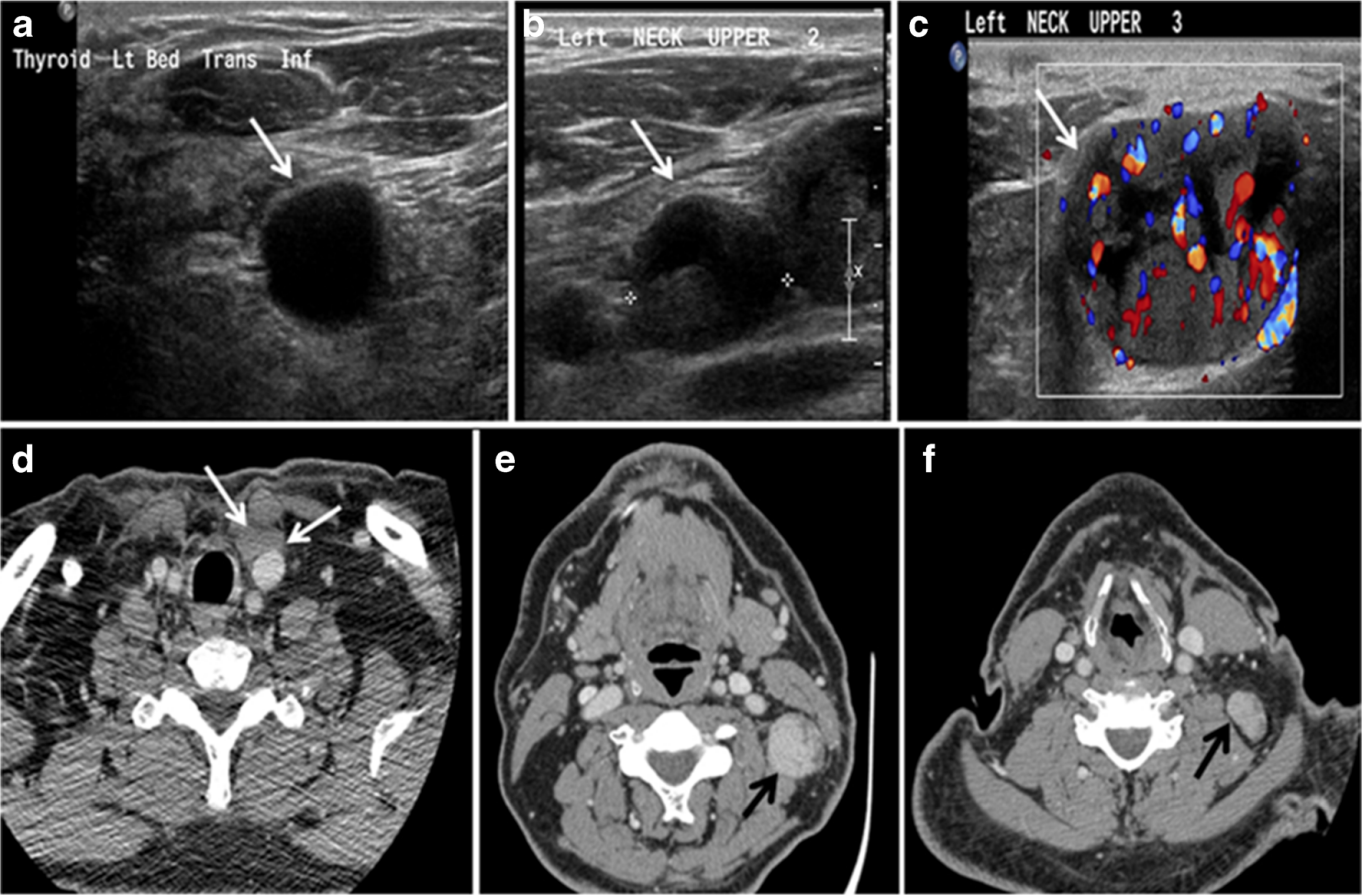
A 58-year-old male patient with persistence PTC at thyroid bed with hypervascular nodal metastasis. a–c Transverse greyscale and colour Doppler neck ultrasound demonstrate hypoehoic soft tissue in the left thyroid bed (*white arrow* in a). There are a heterogeneous enlarged lymph nodes at level 2 and 3 with markedly increased vascularity (white arrow in b and c). d–f Enhanced axial CT images of the neck demonstrate a 2.7 × 1.4 cm hypodense soft tissue lesion anterior to the left carotid sheath (*white arrow*). There are left-sided enhancing abnormal and enlarged lymph nodes at cervical level 2 and 3 (*black arrows*)
